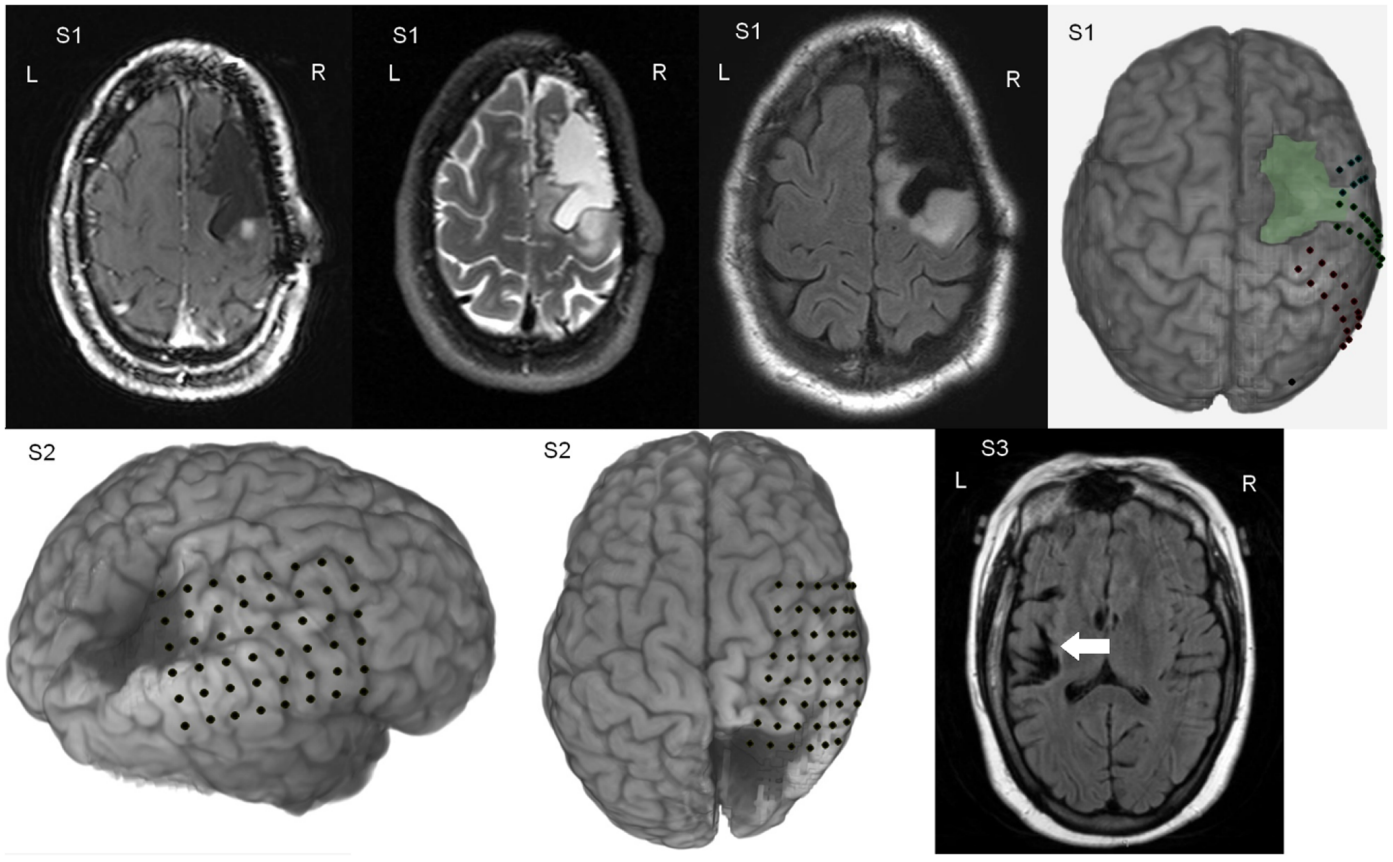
Presurgical MRI and brain reconstructions. Reconstructions are shown for subject 1 (first row) subject 2 (bottom left and middle) and subject 3 (bottom right). The previous resection margins anterior to the precentral gyrus in subject 1 are highlighted in green in the upper right. Superior oblique and top axial views of the reconstruction for subject 2 show the lesion from different viewpoints. Pre-surgical MRI (FLAIR) of subject 3 reveals a lesion of posterior left insula also involving left internal capsule.
